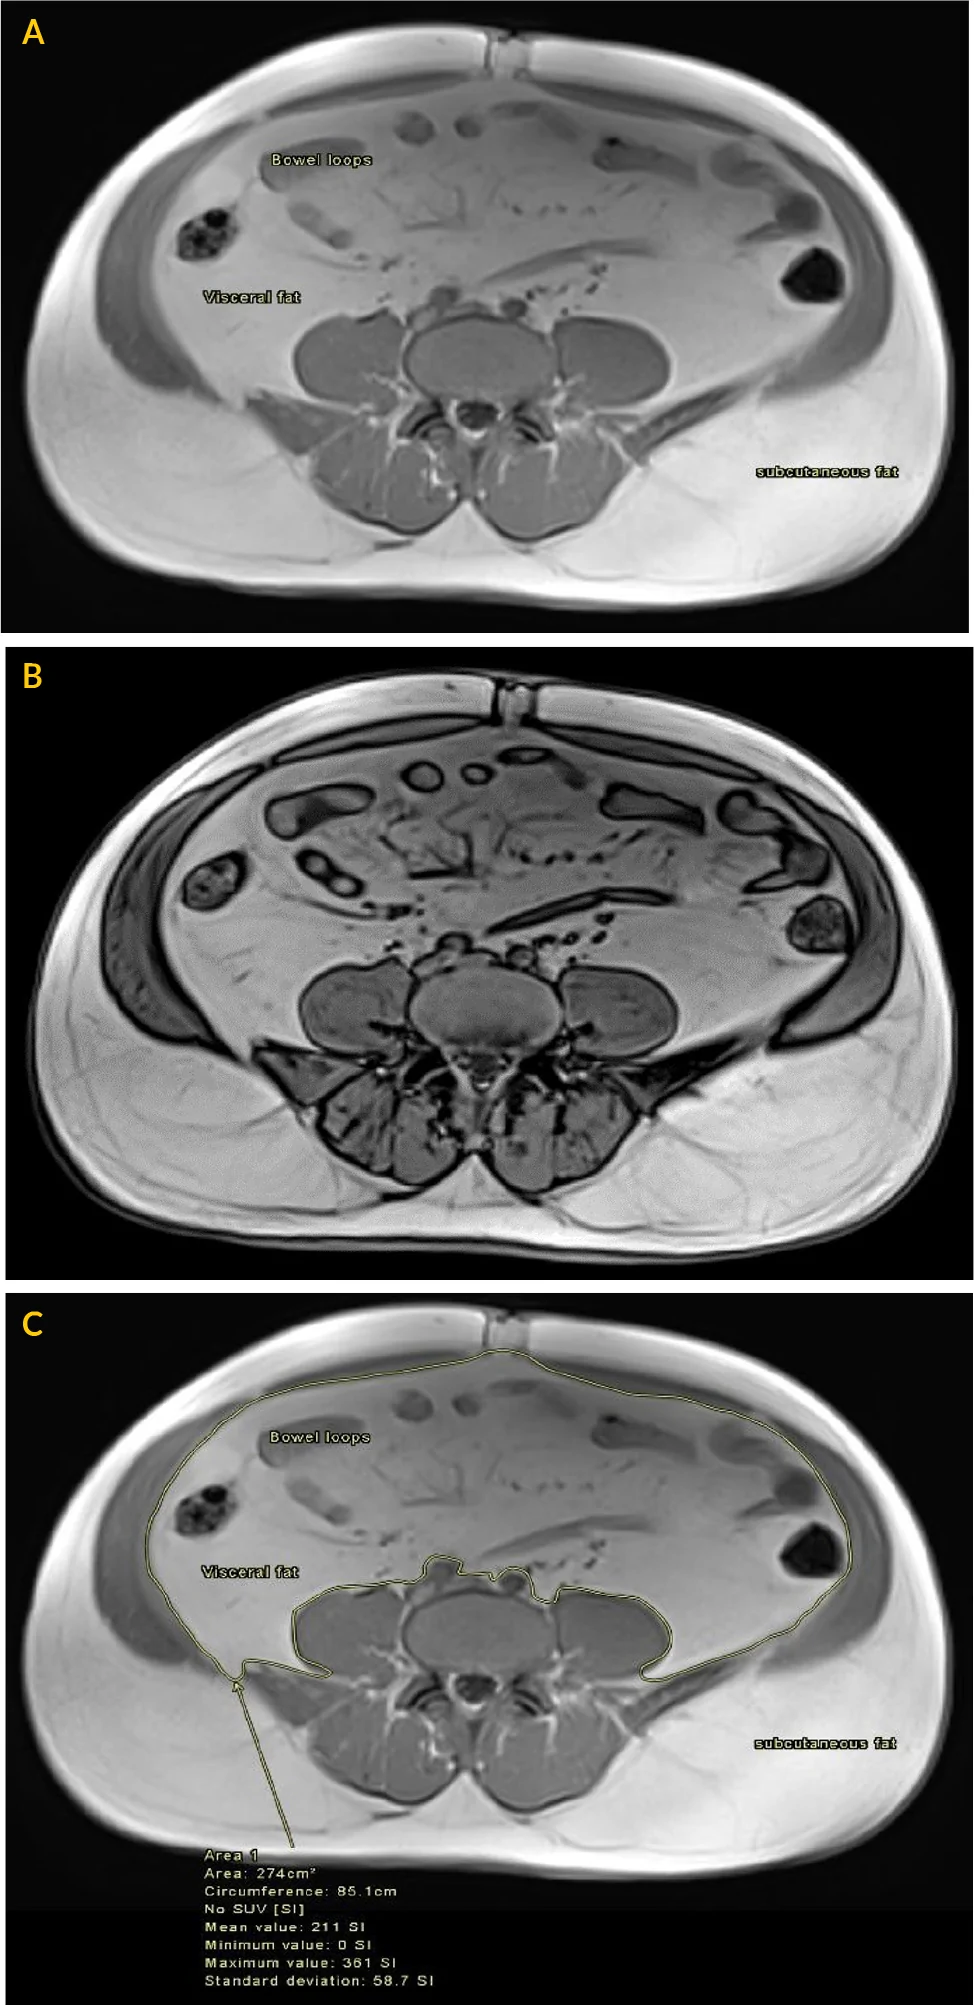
Axial abdominal MRI at the L4-L5 level, depicting visceral fat
